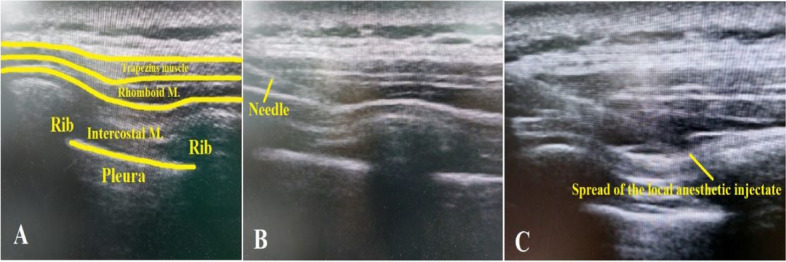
The rhomboid block technique: (A) Sonographic anatomy of the layers encountered during rhomboid block. (B) Directing the needle to the plane between intercostal and rhomboid muscles. (C) Spread of the local anesthetic injectate in the proper plane
